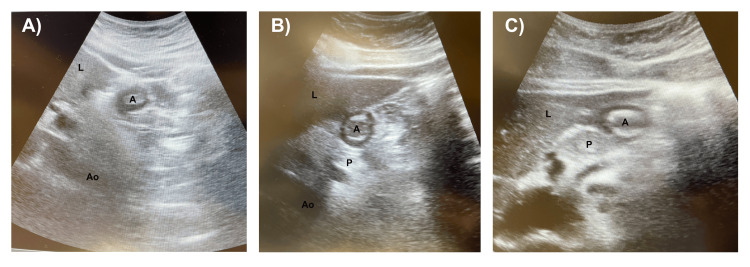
Gastric point-of-care ultrasound demonstrating the empty stomach of three different patients (A, B, and C) The images of these patients were obtained in the right lateral decubitus position to increase the sensitivity of the exam. Pertinent structures were identified, such as the liver (L), gastric antrum (A), aorta (Ao), and pancreas (P). An empty stomach has a “bulls-eye” appearance, indicative of a small or collapsed antrum with a distinct circumferential stomach wall, as seen in A, B, and C.
